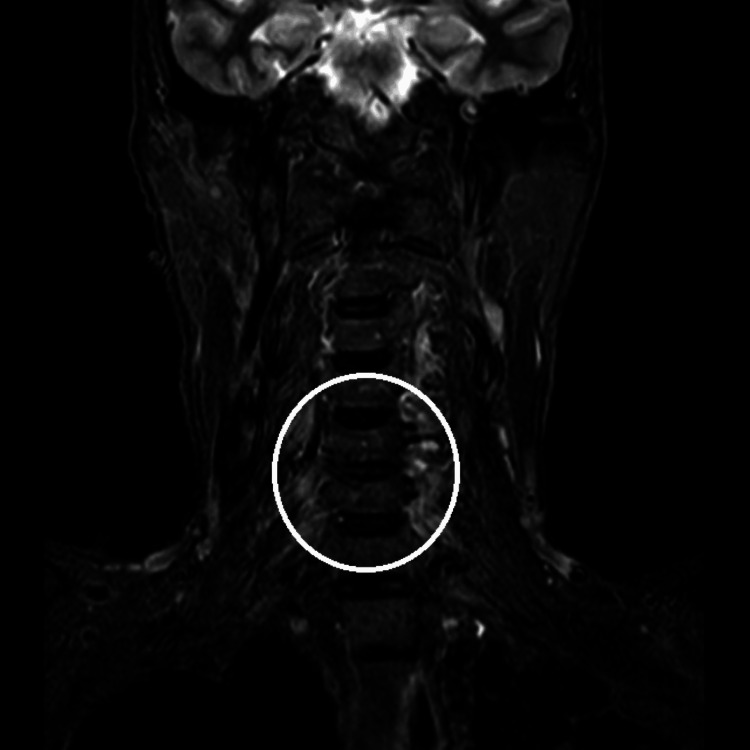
MRI cervical spine MRI: Magnetic resonance imaging The circle denotes C3-C4 diffuse disc bulge indenting over the anterior thecal sac, causing mild narrowing of the neural foramina indenting over the exiting nerve roots.
